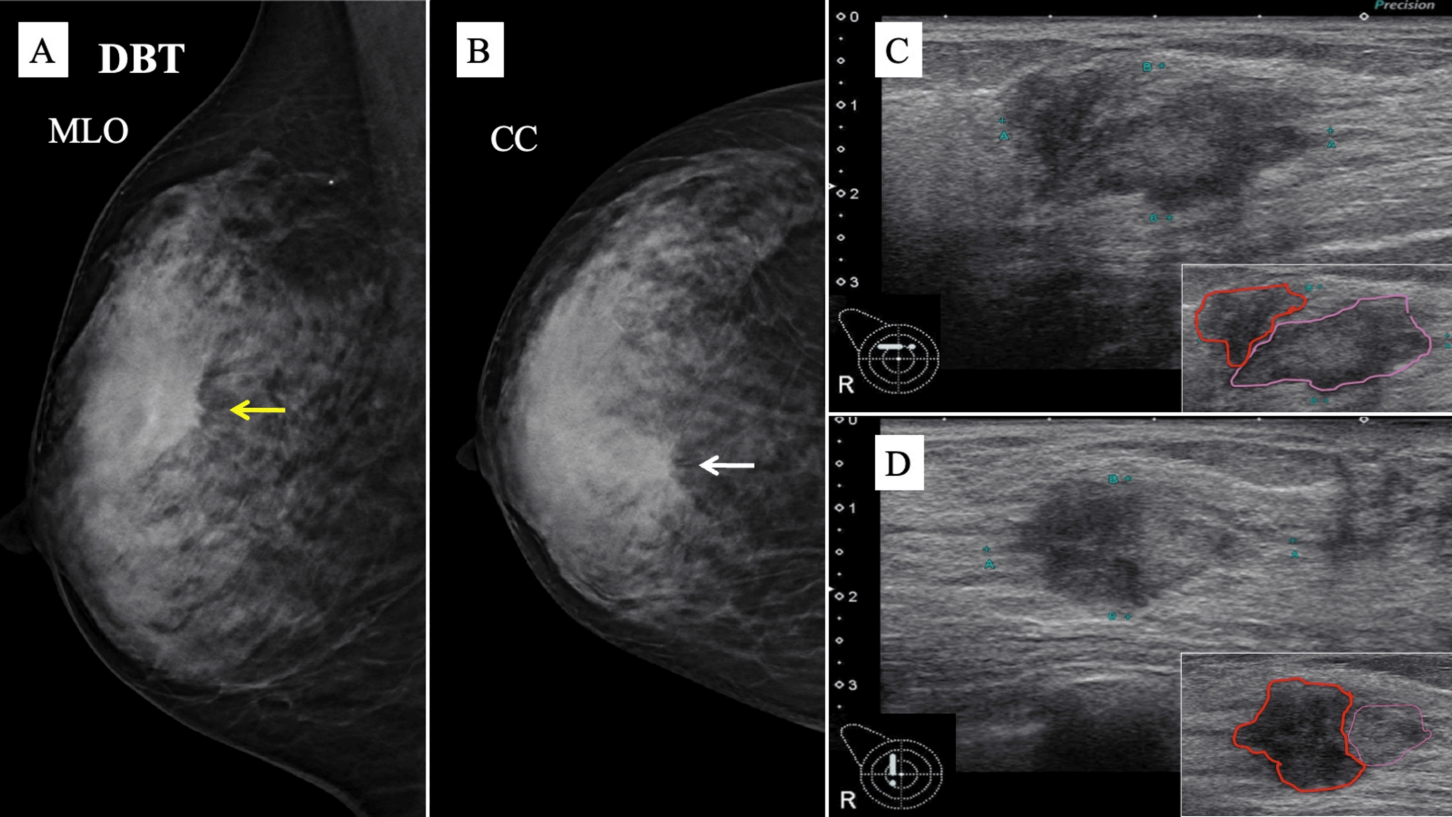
MG and US findings. (A) DBT shows a high-density spiculated mass (yellow arrow) on the MLO view. (B) A CC view of DBT reveals architectural distortion surrounding the mass (white arrow). (C, D) US findings. The transverse US image in C reveals two adjacent irregular hypoechoic masses. The internal echoes were slightly different in the longitudinal view, as shown in D. The tumor margins are highlighted in pink and red in the lower right insets of C and D, respectively. The pink-outlined tumor is HER2-negative, and the red-outlined tumor is HER2-positive breast cancer. Tumors outlined in red have lower internal echo values. CC: craniocaudal; DBT: digital breast tomosynthesis; HER2: human epidermal growth factor receptor 2; MG: mammography; MLO: mediolateral oblique; US: ultrasonography
